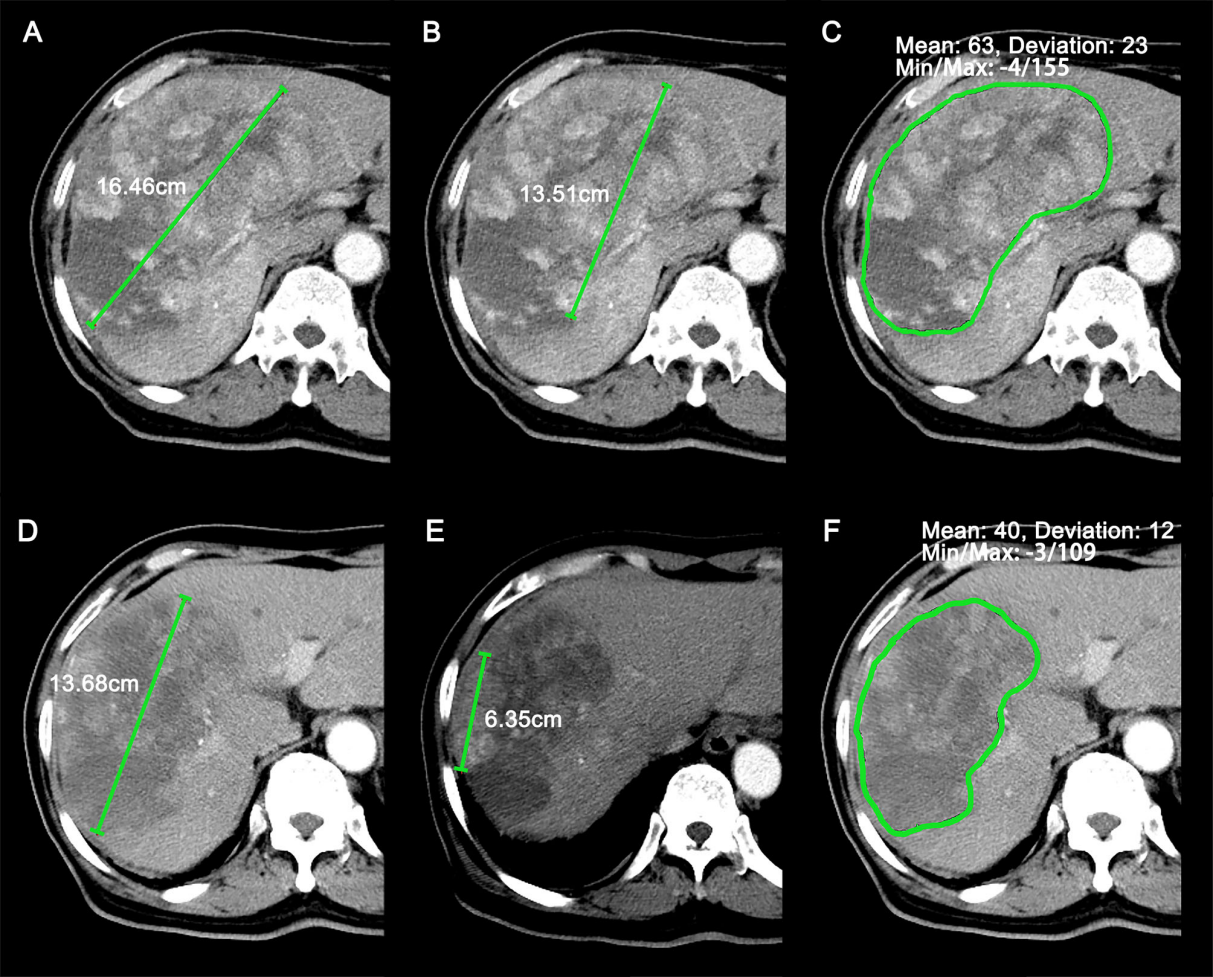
Representative case (69-year-old male patient) of target lesions measured at baseline and the first follow-up scan. (A–C) Sum of longest diameters (SLDs) (A), SLDs of viable (enhancing) target lesions (B), and average attenuation of the target lesions (C) at baseline CT images. (D–F) SLDs (D), SLDs of viable (enhancing) target lesions (E), and average attenuation of the target lesions (F) at the first follow-up CT images. The patient was identified as stable disease (SD), partial response (PR), PR, and PR by RECIST 1.1, modified RECIST (mRECIST), Choi, and revised Choi (rChoi), respectively.
